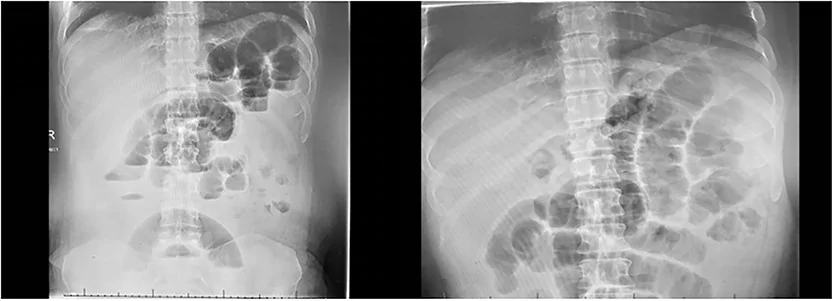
The abdominal X-ray showed an air–fluid level with a dilated small bowel.
